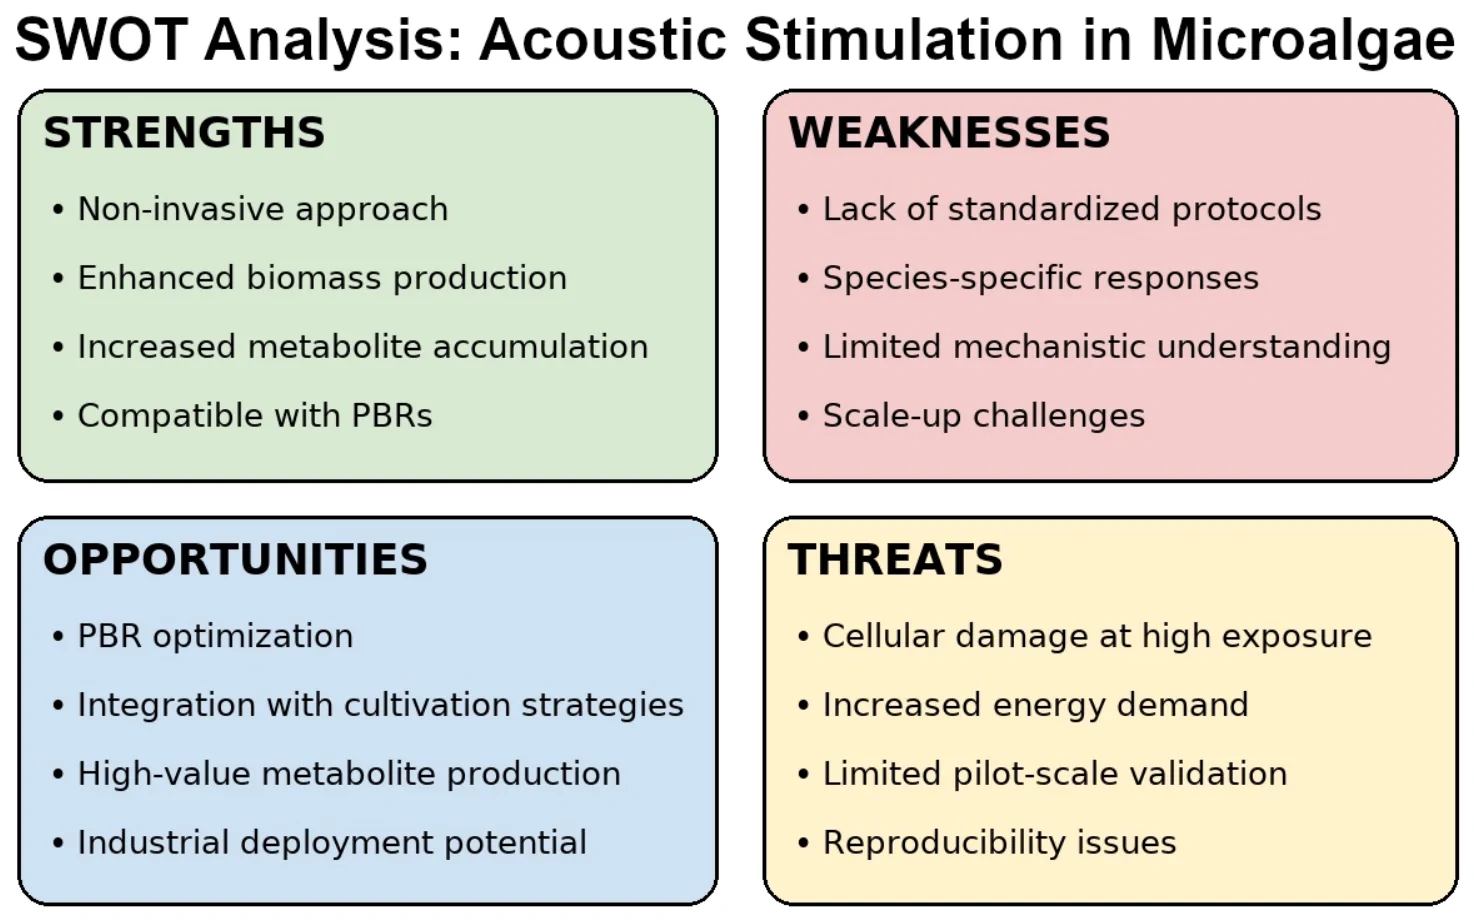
SWOT analysis of audible sound and ultrasound applications in microalgal biotechnology.
